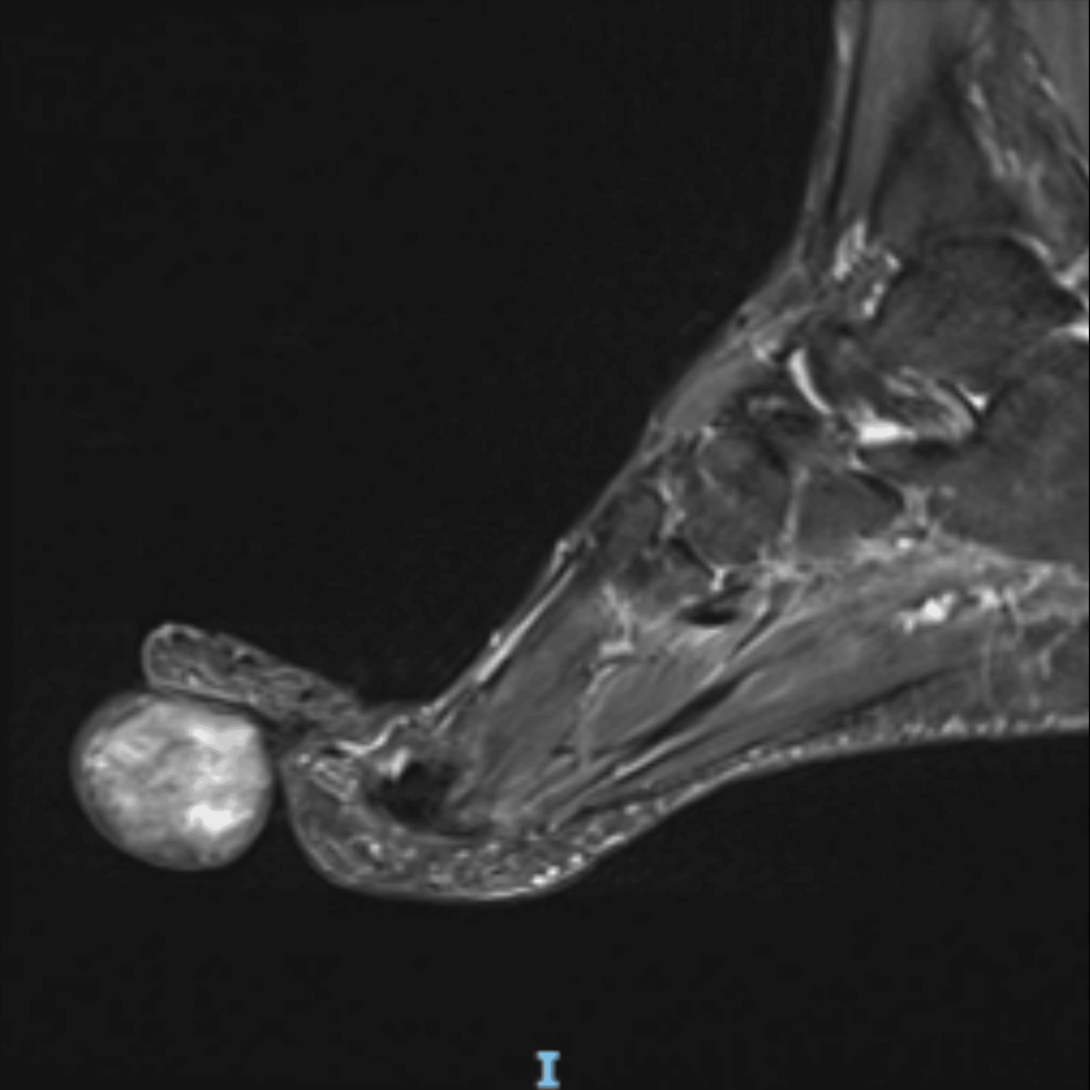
MRI imaging (sagittal section). T2-weighted images showing heterogenous hyperintensity signals.
